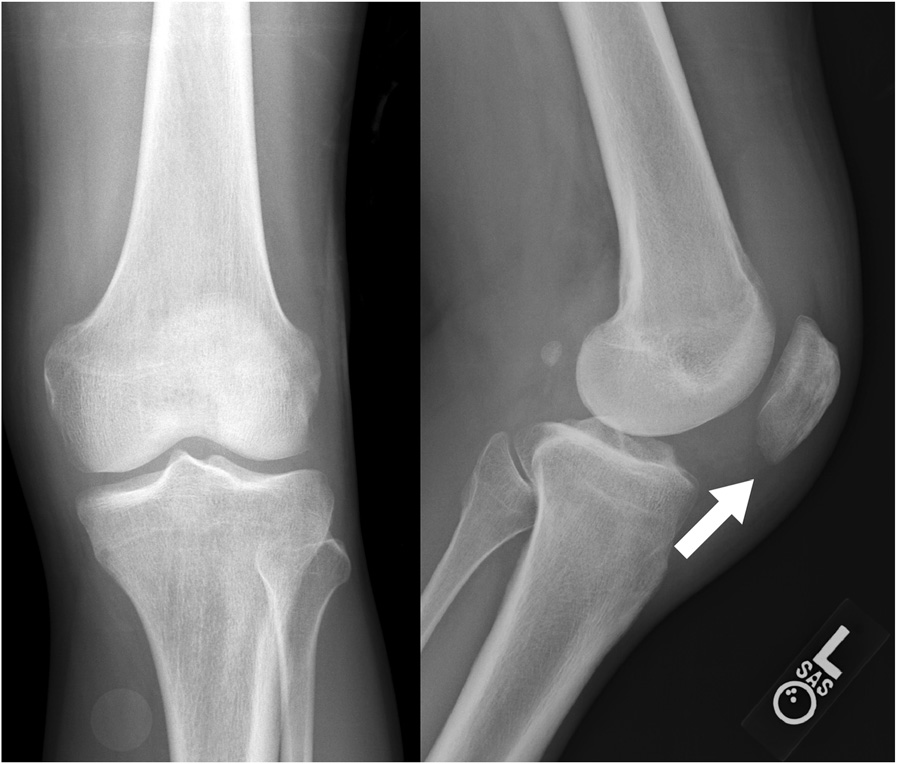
AP and lateral radiographs of the left knee demonstrate lucency involving the inferomedial patella with questionable focal lesion (white arrow) and a joint effusion. No soft tissue calcification is present.
